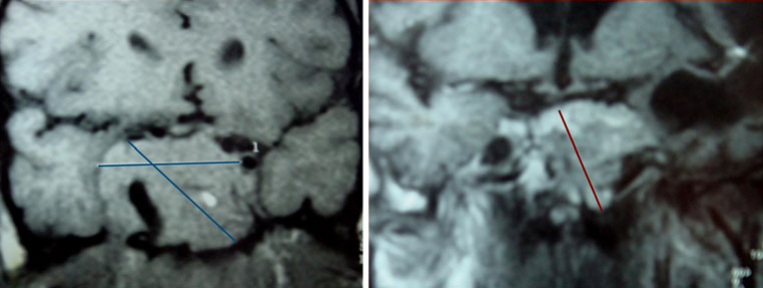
MRI scans of pituitary tumor at diagnosis (December 1994, *left*) and 9 years after surgery and radiotherapy without medical treatments (January 2008, *right*)
